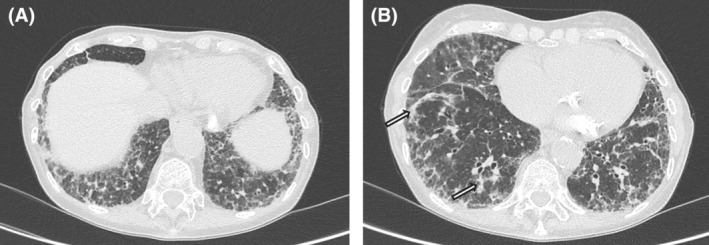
(a) Lung HRCT, July 2023: The extent of disease is increased. (b) Some perilobular bands of consolidations, resembling organising pneumonia, are appreciable (white arrows).
